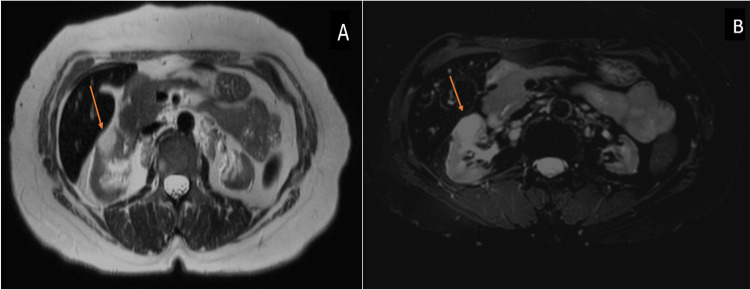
A & B: Axial T2 and T2 fat sat MRI images of the abdomen showing a hyperintense mass of the right kidney (arrow). The left kidney is atrophic.
